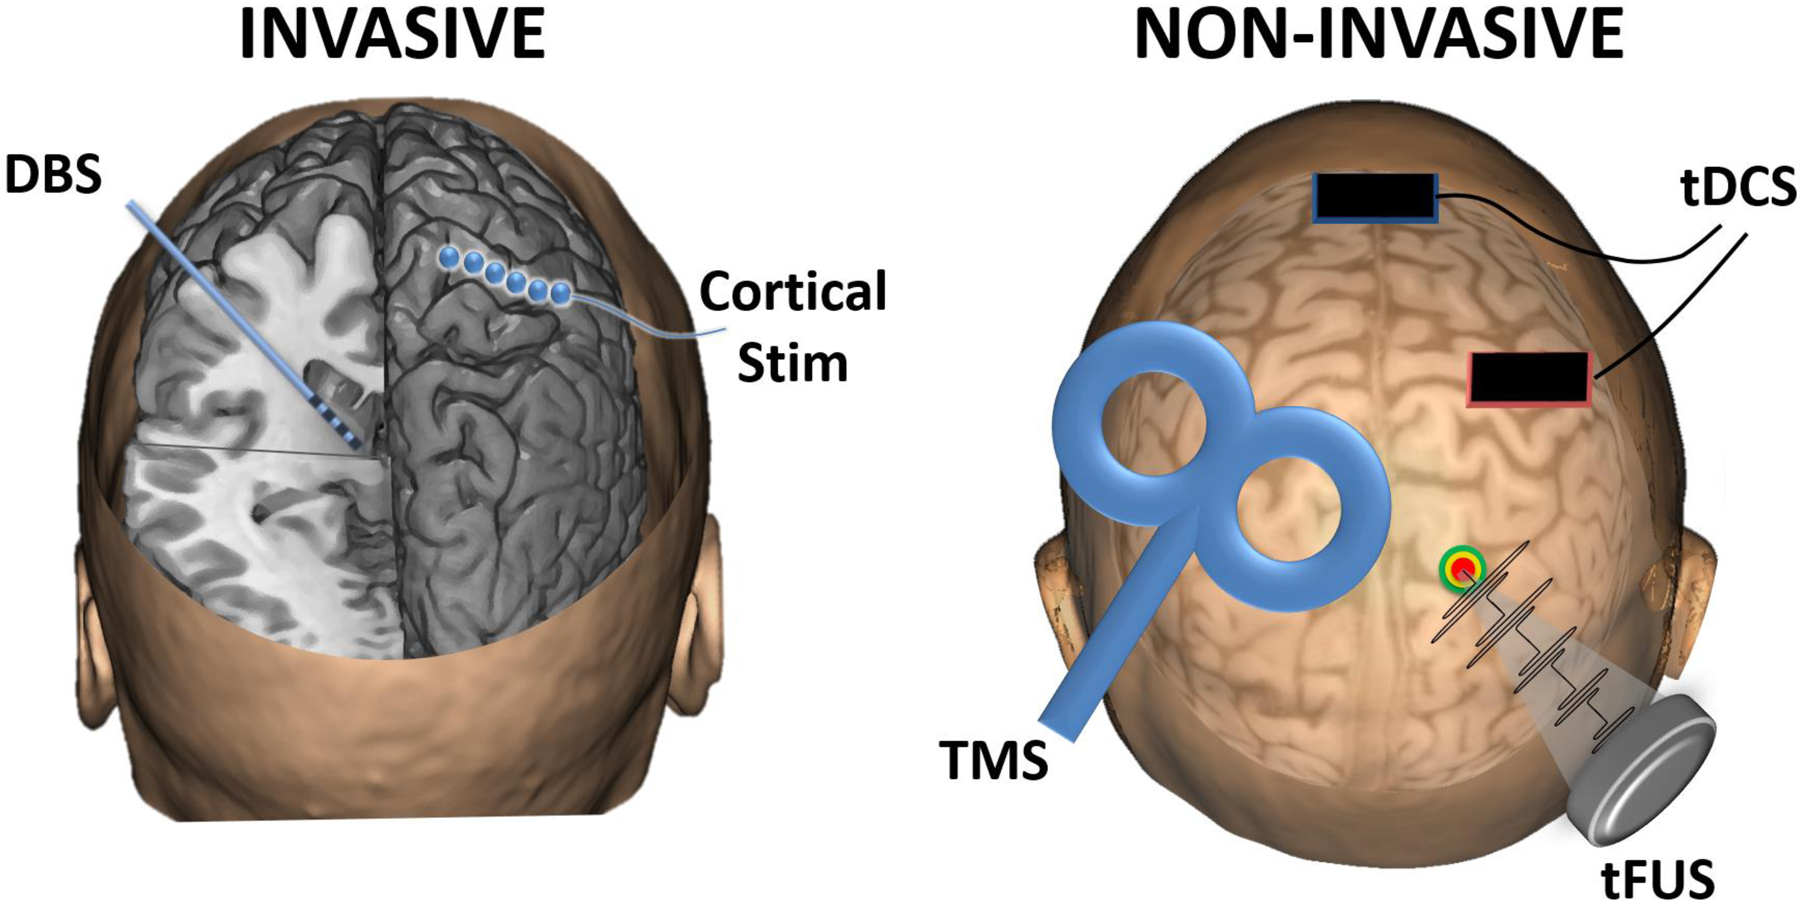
A summary of invasive and noninvasive neuromodulation technologies. Invasive techniques include DBS, in which a lead is implanted into a deep brain structure, and cortical stimulation, in which electrodes are placed on the brain surface. Noninvasive techniques include transcranial magnetic stimulation (TMS), typically applied with a figure-eight coil, transcranial direct-current stimulation (tDCS) via scalp sponge electrodes, or transcranial focused ultrasound stimulation (tFUS) using pulsed ultrasound from a transducer on the scalp.
